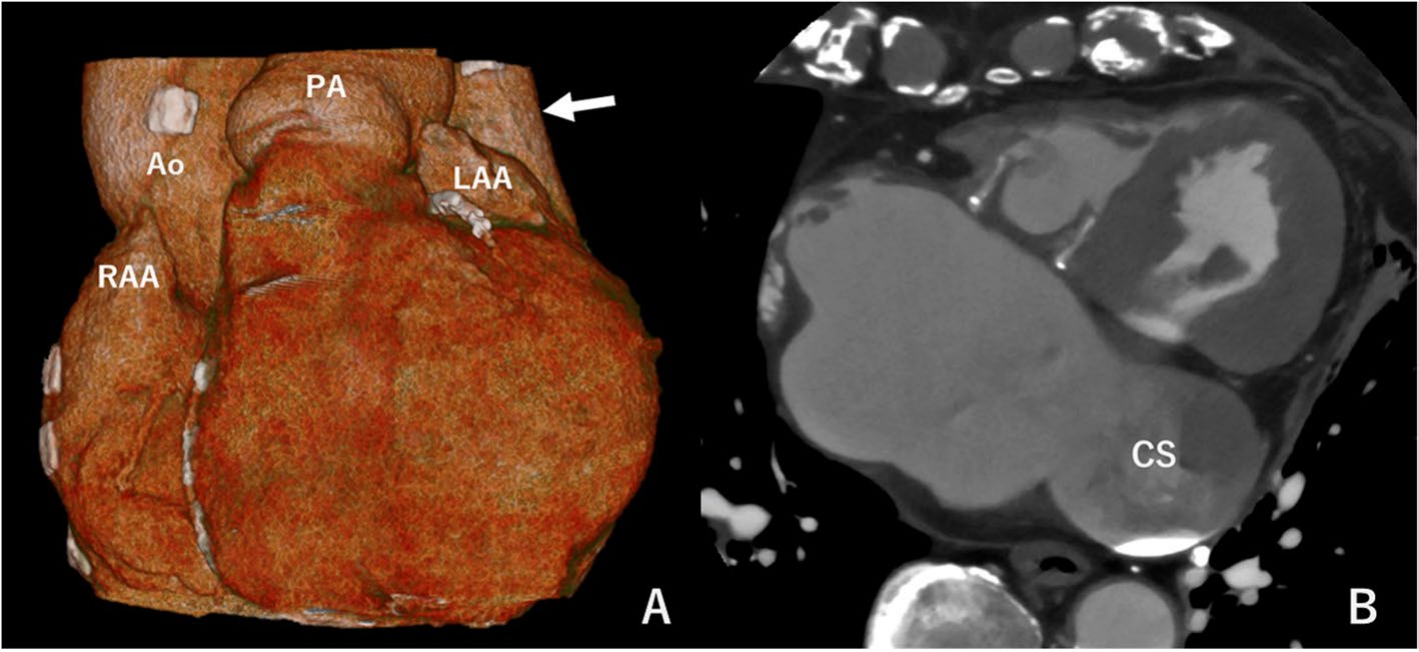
A Three-dimensional computed tomography image shows persistent left superior vena cava (white arrow). B Axial image shows enlarged coronary sinus (maximum diameter, 42 mm). *Ao* aorta, *CS* coronary sinus, *LAA* left atrial appendage, *PA* pulmonary artery, *RAA* right atrial appendage
